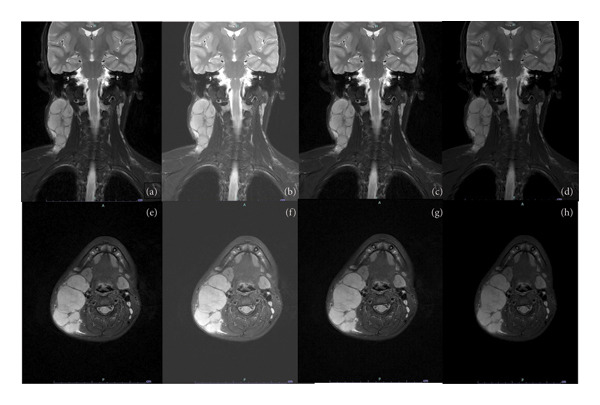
Radiographic features of RDD: (a) coronal original image, (b) coronal head FLAIR image, (c) coronal T1 image, (d) coronal T2 image, (e) axial original image, (f) axial head FLAIR image, (g) axial T1 image, and (h) axial T2 image.
